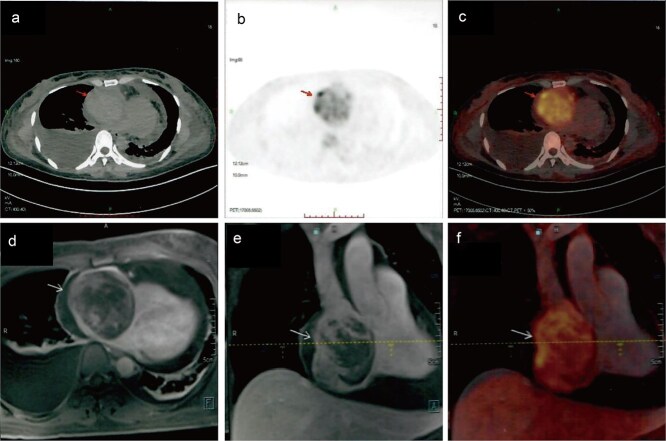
Metabolic imaging. PET-CT (a) and (b) and PET-MR (c) and (d) demonstrated a hypermetabolic soft tissue mass within the right atrium, highly suggestive of primary cardiac angiosarcoma.
